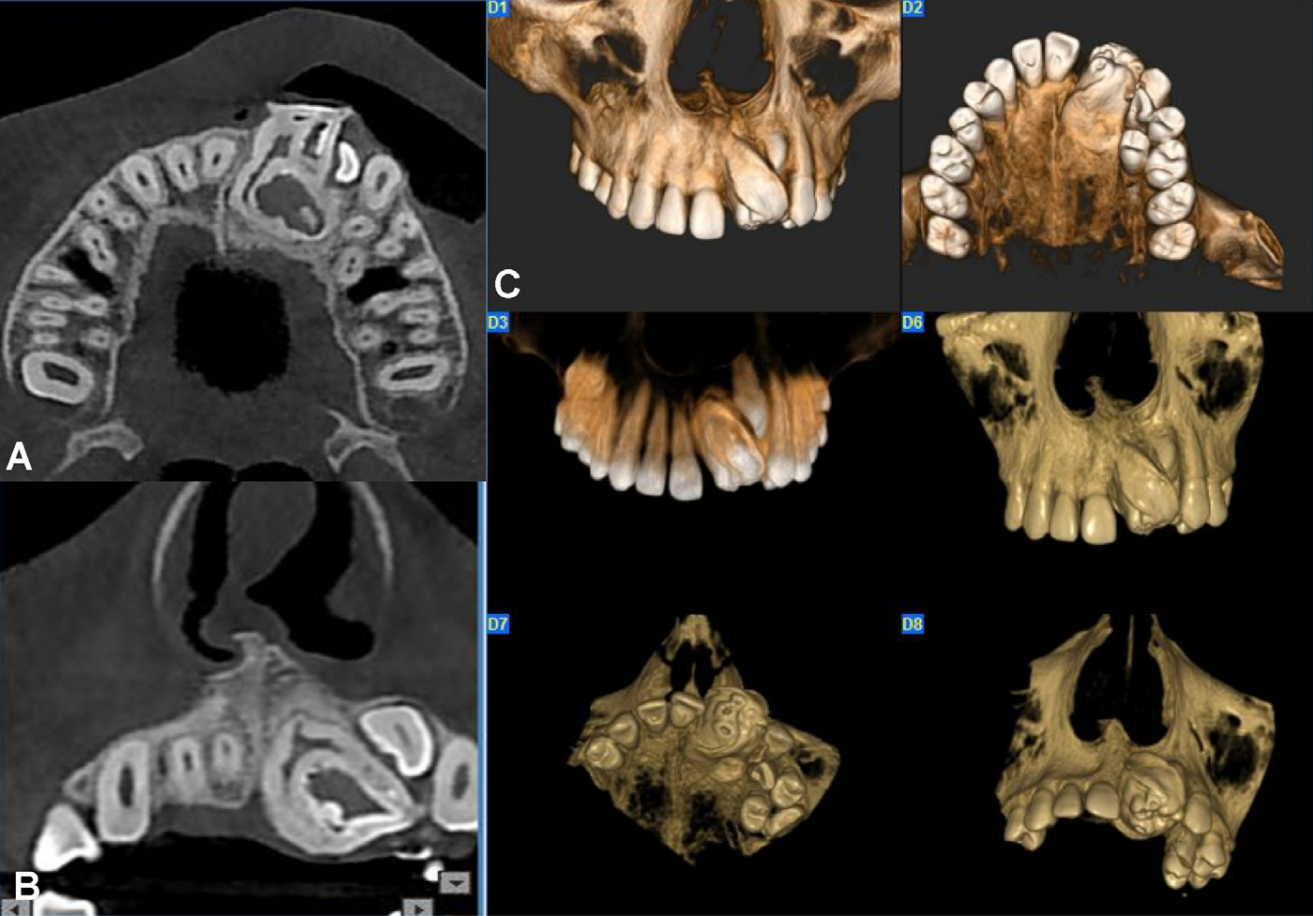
Cone beam computed tomography images showing a large compound odontoma with thin radiolucent rim surrounding the mineralized tissue, and interference with the eruption of associated teeth.: (A) axial view, (B) coronal view, and (C) three-dimensional reconstruction of tumor.
